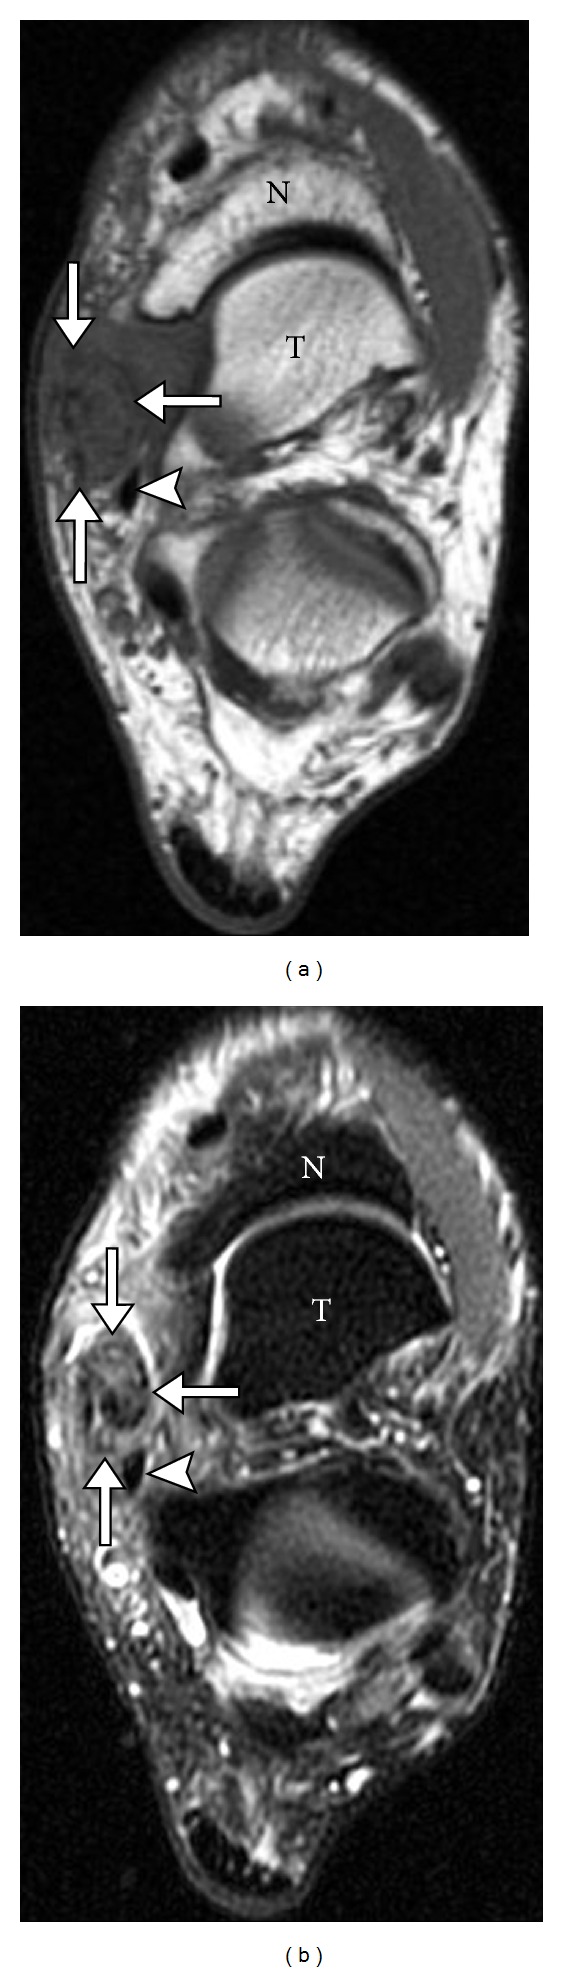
Gout involving tibialis posterior tendon. Axial T1 (a) and axial T2 (b) MR images show abnormal soft tissue infiltrating and surrounding the tibialis posterior tendon (arrows) adjacent to flexor digitorum tendon (arrowhead). Patient subsequently underwent surgery revealing complete rupture secondary to gout.
